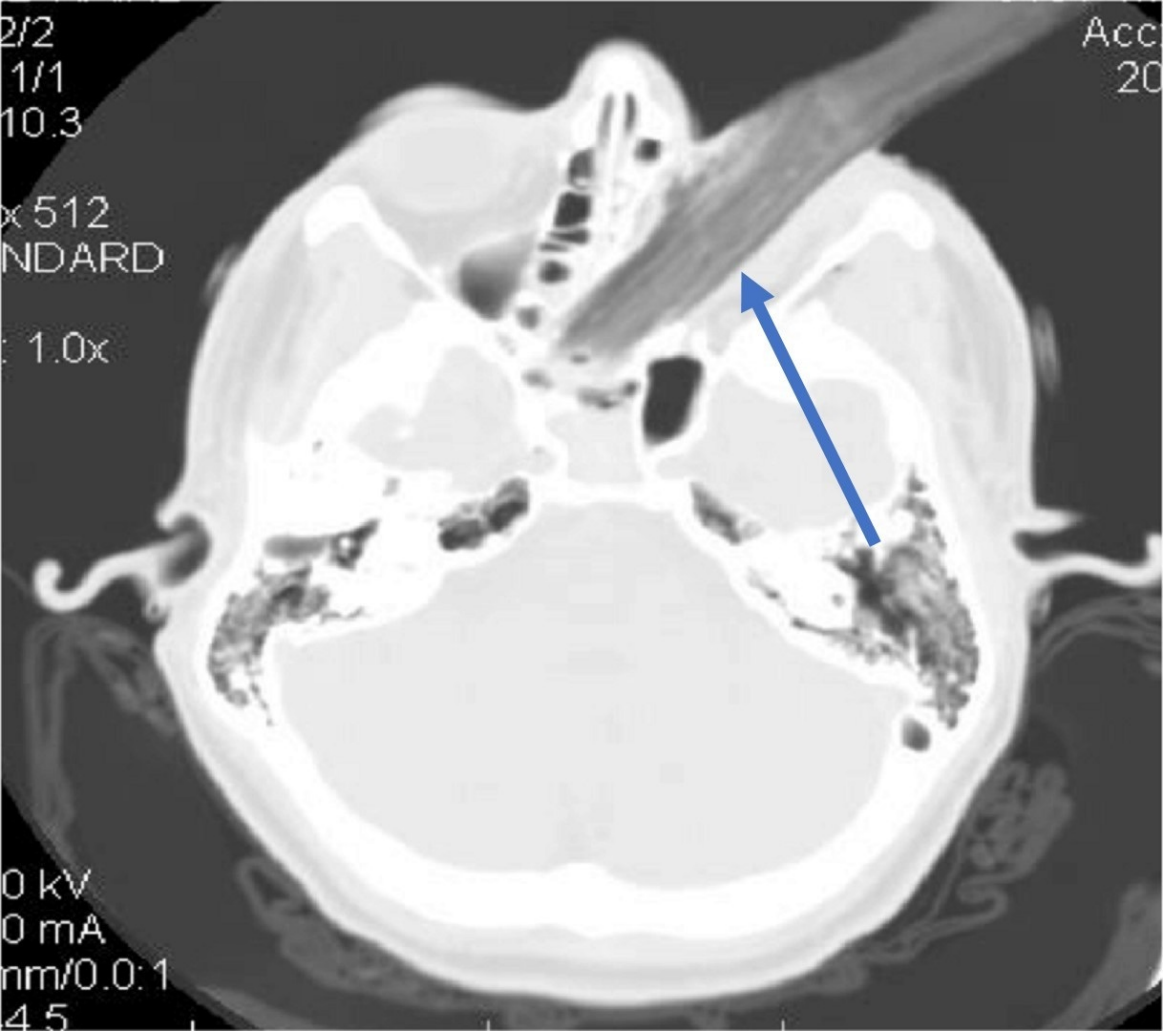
Computed tomography (CT) of head: axial view through the orbit. Blue arrow: tree branch in the left orbit extending into the nasal cavity, ethmoidal air cells, and right sphenoid sinus via the medial orbital wall.
